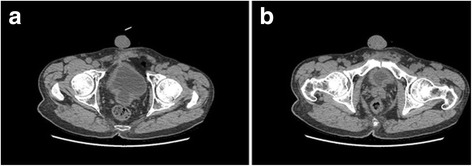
Computed tomography (CT) of the abdomen and pelvis. A, the bladder wall was thickened; B, the neck of bladder was thicker than the bladder wall
